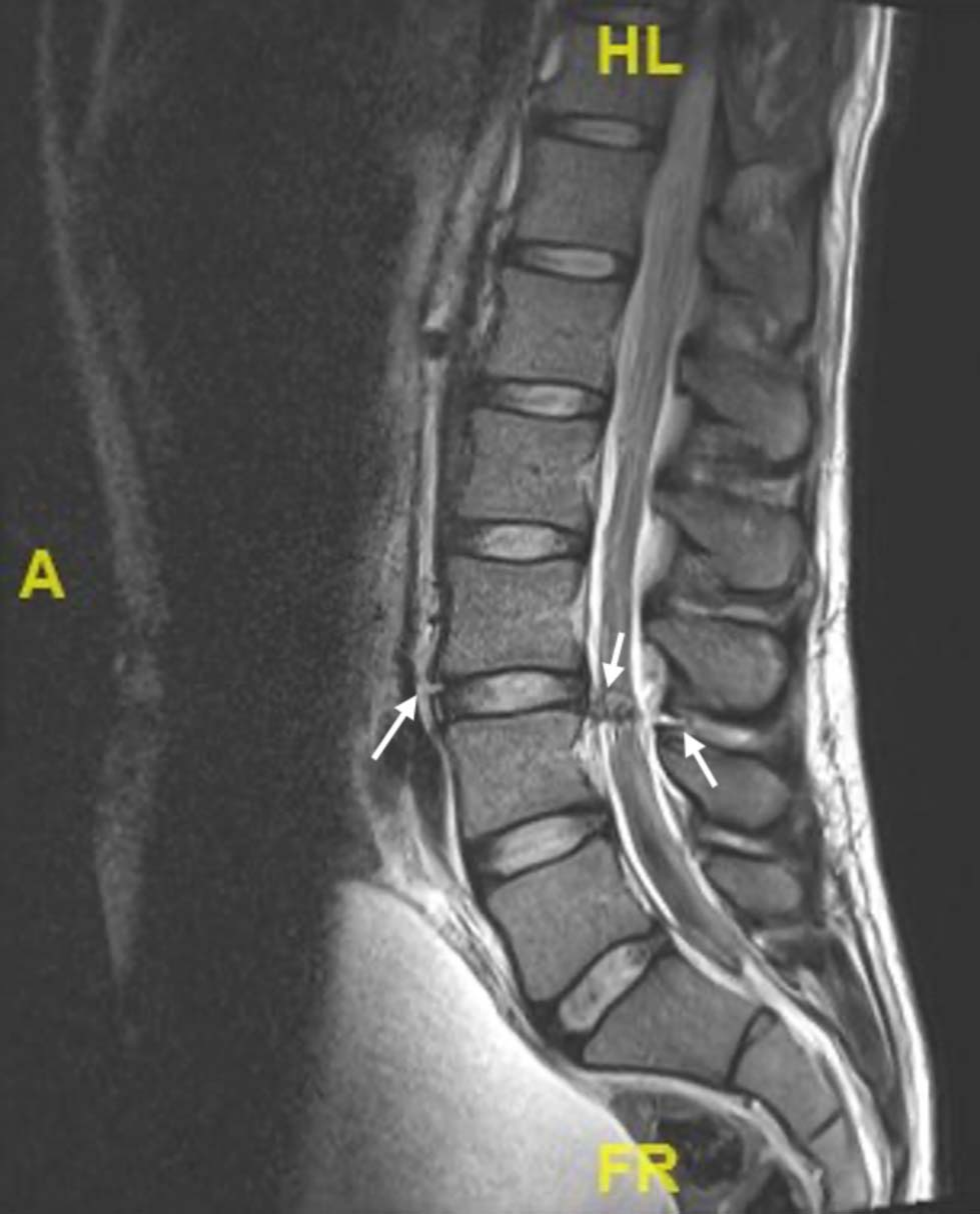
Sagittal MRI cut showing reported disk extrusion. Arrows demonstrate disruption of the anterior longitudinal ligament.
